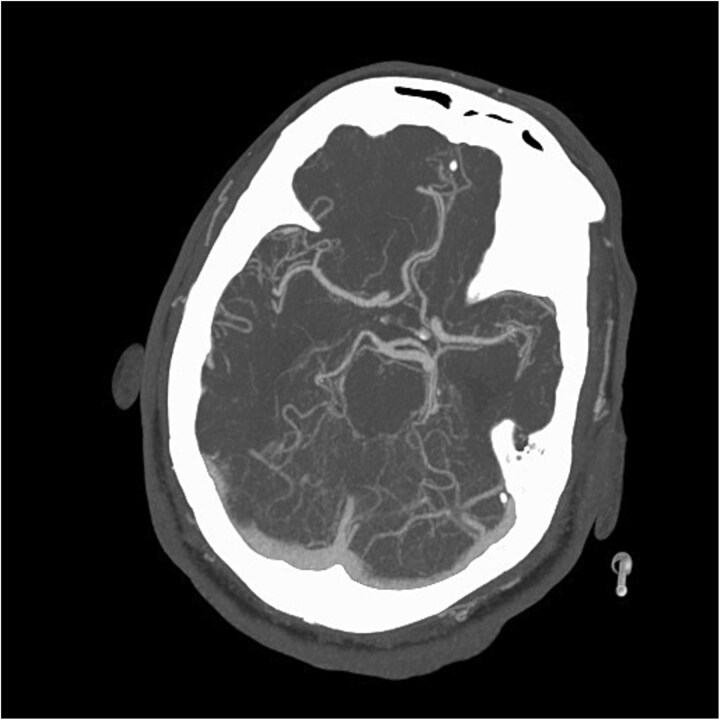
Repeat CTA head showing improved caliber of the anterior and posterior cerebral arteries following Milrinone treatment.
